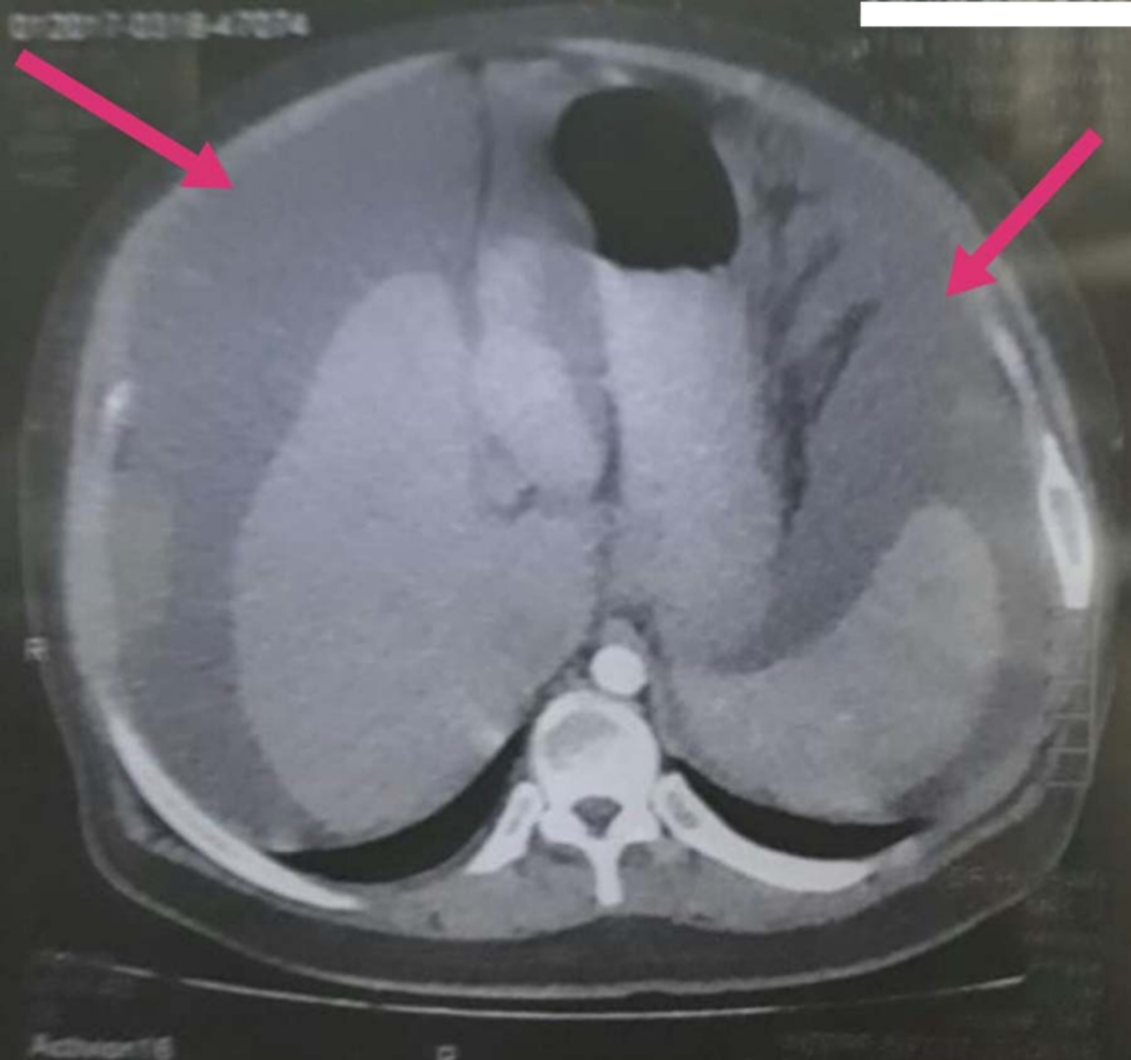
Axial view CT abdomen, periphery showing ascites CT: Computed tomography
